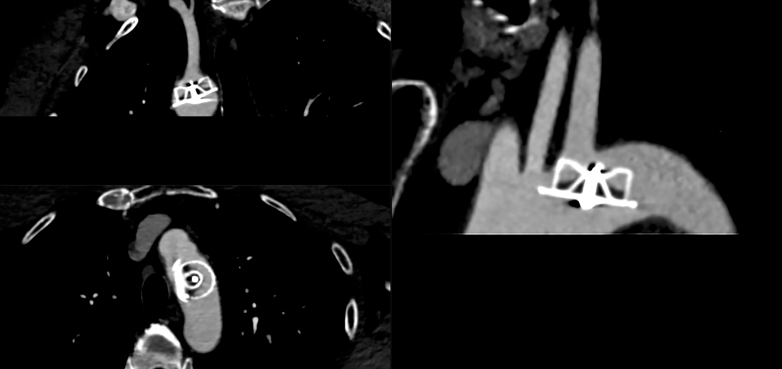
Computed Tomography Angiogram of Aortic Arch Computed tomography angiogram demonstrated the presence of the embolized Amulet left atrial appendage occlusion device in the transverse aorta at the left subclavian artery ostium.
